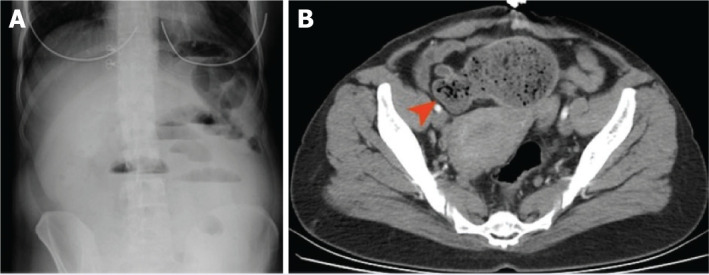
Plain abdominal radiography (A); air-fluid levels consistent with mechanical intestinal obstruction, abdominal computed tomography examination (B); oval-shaped lesion in the small intestine lumen at the level of the ileum, containing air beads (compatible with bezoar), causing obstruction.
